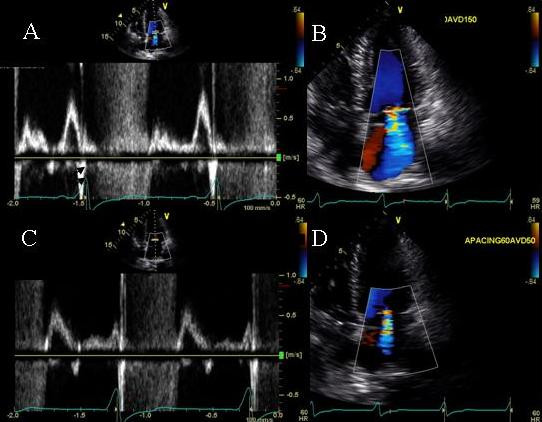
Figure shows the effect of AVD on mitral regurgitation severity. Mitral inflow pulsed Doppler (A) and color Doppler showing mitral regurgitation in the apical 4 chamber view (B) at a paced AV delay of 150 ms and mitral inflow pulsed Doppler (C) and color Doppler showing mitral regurgitation severity in the apical 4 chamber view (D) at paced AV delay of 50 ms. Diastolic mitral regurgitation is seen at an AV delay of 150 ms (black arrowheads, A). Note significant mitral regurgitation in panel B which decreased in panel D at short AV delay.
